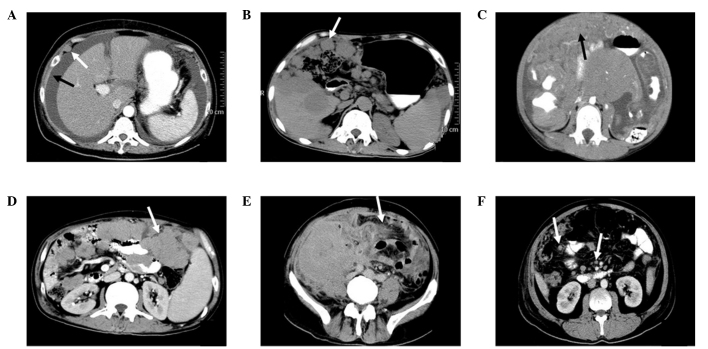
CT scans of non-Hodgkin lymphoma cases with peritoneal, omental or mesenteric involvement. (A) Diffuse large B-cell lymphoma with peritoneal involvement (58-year-old female patient), exhibiting peritoneal nodular thickening (white arrow), cord-like thickening (black arrow) and a significantly thicker peritoneum. Diffuse large B-cell lymphomas with omental involvement, exhibiting (B) peritoneal nodular thickening (white arrow; 48-year-old male patient), (C) omental cake-like thickening (black arrow; 7-year-old male patient) and (D) omental tumor-like thickening (white arrow; 36-year-old male patient). (E) T-cell lymphoma (33-year-old male patient), exhibiting an increased intestinal gap fat density (white arrow). (F) Follicular lymphoma with mesenteric involvement (40-year-old male patient), exhibiting nodules in the mesentery and mesenteric roots (white arrows).
